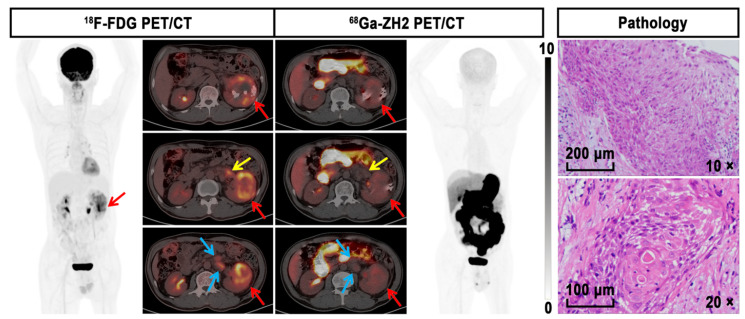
Comparative ^18^F-FDG and ^68^Ga-ZH2 PET/CT images in patient #19 with left renal keratinizing squamous cell carcinoma. The primary renal tumor (red arrows), left renal vein tumor thrombus (yellow arrows), and retroperitoneal lymph node metastasis (blue arrows) are all ^18^F-FDG-avid but demonstrate no uptake on ⁶⁸Ga-ZH2 PET/CT.
